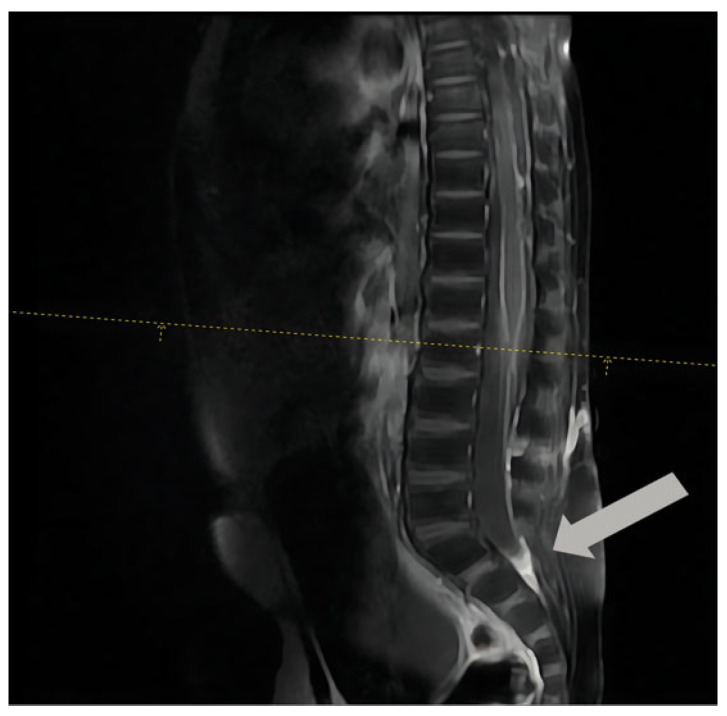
Magnetic Resonance Imaging shows distinct leptomeningeal enhancement along the lumbar spine and cauda equina nerve roots, as indicated by an arrow.
